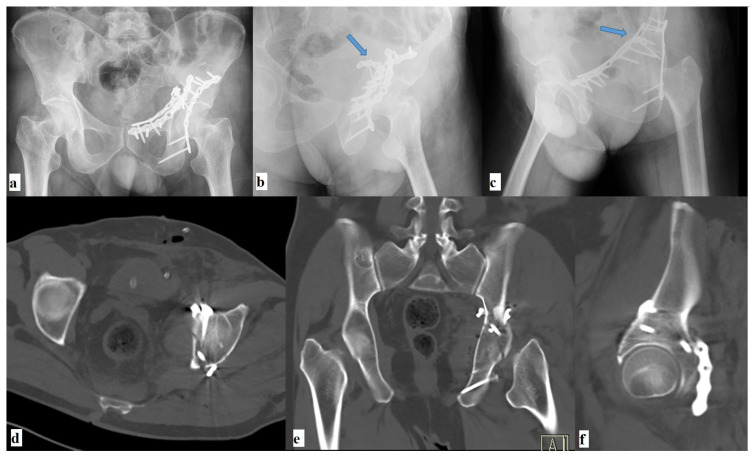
(a,b,c) Postoperative radiographs and (d,e,f) CT images of the same patient. The blue arrows show the screws placed using the 105-degree drill attachment.
